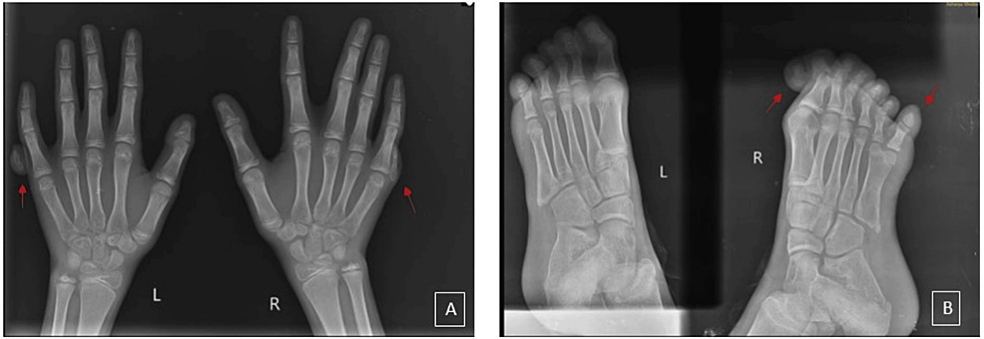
Case 1: X-rays of hands and feet confirming polydactyly
